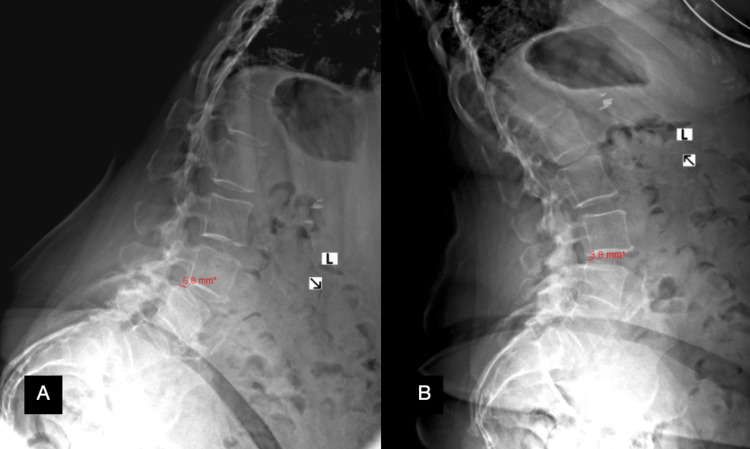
Flexion (A) and extension (B) X-ray of the lumbar spine.
